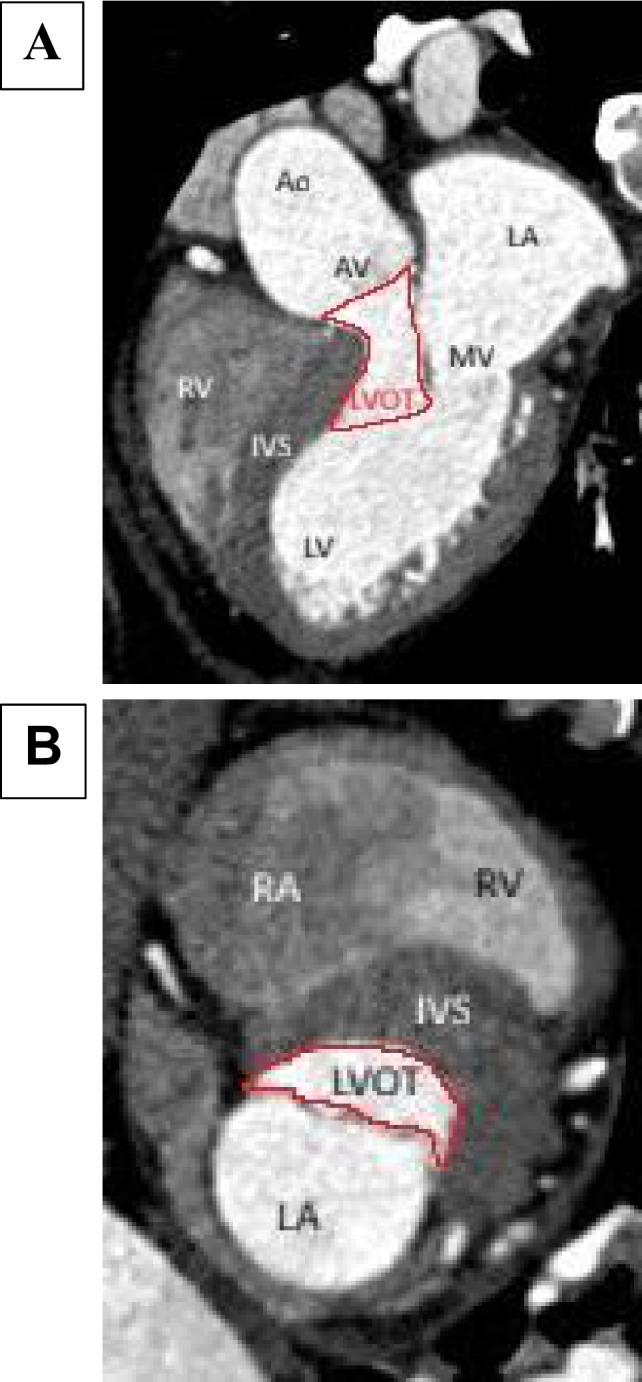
A Boundaries of left ventricular outflow tract on a computed tomography image. B Elliptical shape of left ventricular outflow tract. Ao-Aorta, AV-Aortic Valve, LVOT-Left Ventricular Outflow Tract, LA-Left Atrium, MV-Mitral Valve, LV-LeftVentricle, RV-Right Ventricle, IVS-Inter Ventricular Septum, RA-Right Atrium
